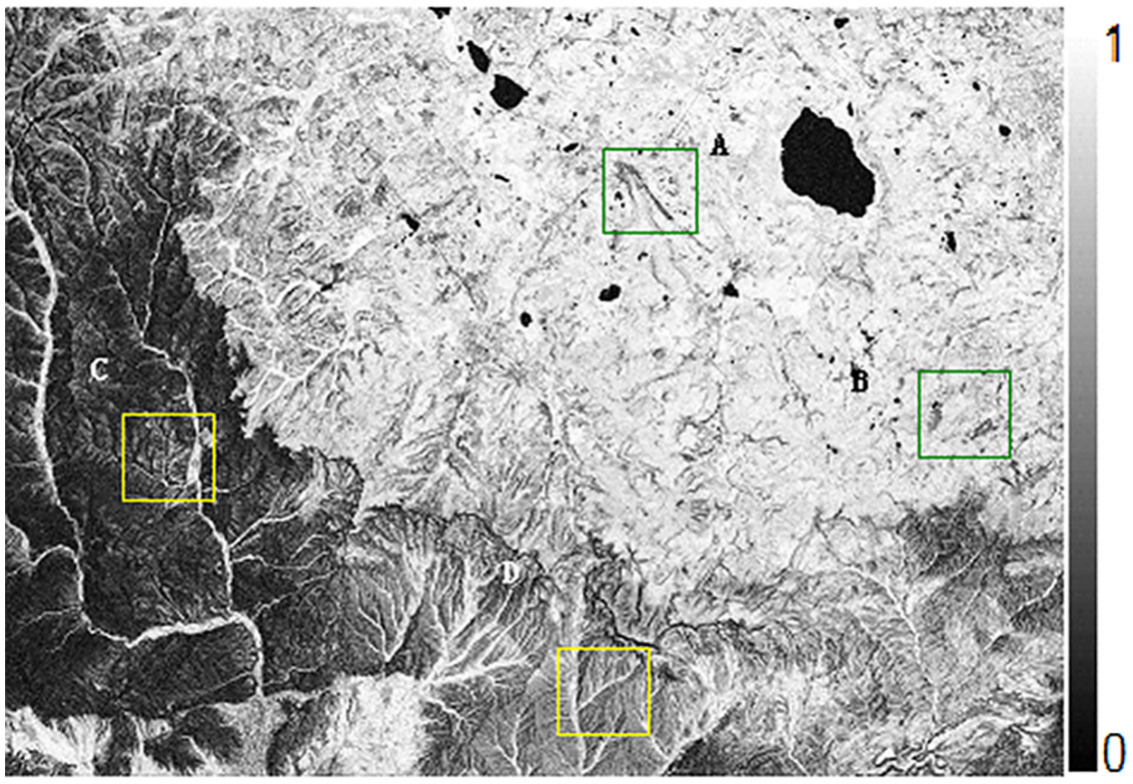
Coherence map from level 0 data with consistent doppler parameters.
